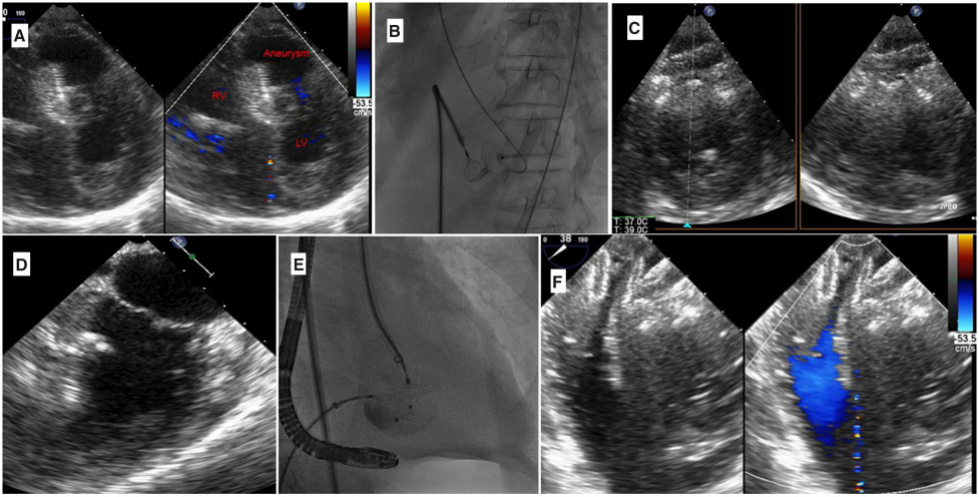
(*A*) Transoesophageal echocardiogram showing an 18-mm Amplatzer septal occluder device used to close the aneurysm to RV communication. (*B*) Fluoroscopy showing the first deployed 18-mm device. (*C*) Transoesophageal echocardiogram transgastric short-axis view showing the 26-mm Amplatzer septal occluder device closing the mouth of the aneurysm. (*D*) Transoesophageal echocardiogram two-chamber view showing the 26-mm Amplatzer septal occluder device closing the mouth of the aneurysm. (*E*) Fluoroscopy showing the two deployed devices connected by cables: one from the femoral vein and the other from the femoral artery. (*F*) Transoesophageal echocardiogram showing the two devices, with the 26-mm device resting on the 18-mm one, no colour flow seen.
